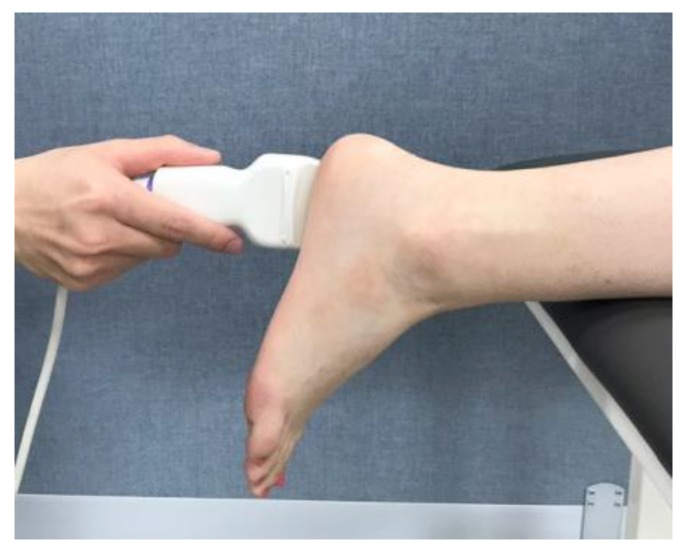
Ultrasonography of plantar fascia.
